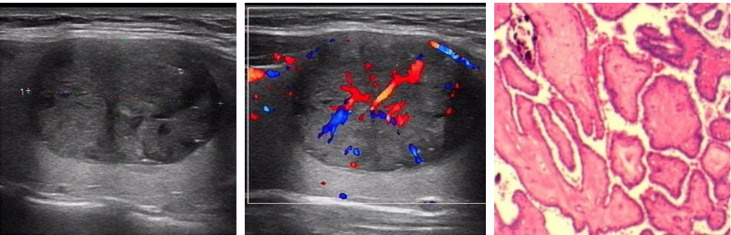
Grayscale ultrasonography shows a solid, hypoechoic nodule in the right lobe of the thyroid with wider-than-tall and smooth margin. It was classified as an ACR TI-RADS 4 lesion with moderate suspicion of cancer requiring biopsy. Color Doppler ultrasonography showed the distribution of spoke wheel blood flow signals, which was determined to be papillary thyroid carcinoma. Postoperative pathology confirmed papillary thyroid carcinoma.
